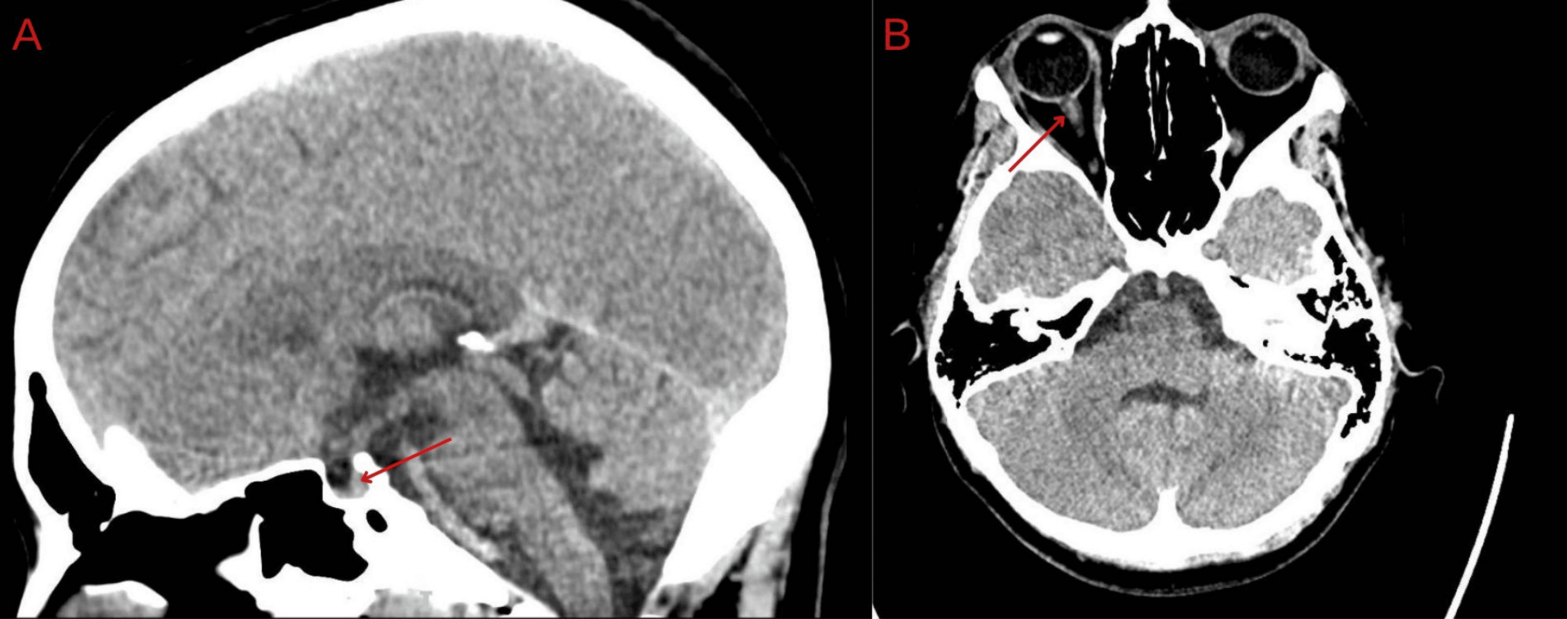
Non-contrast brain CT scan: sagittal view (A) shows a flattened pituitary gland, and axial view (B) demonstrates mild widening of the optic nerve sheaths.
